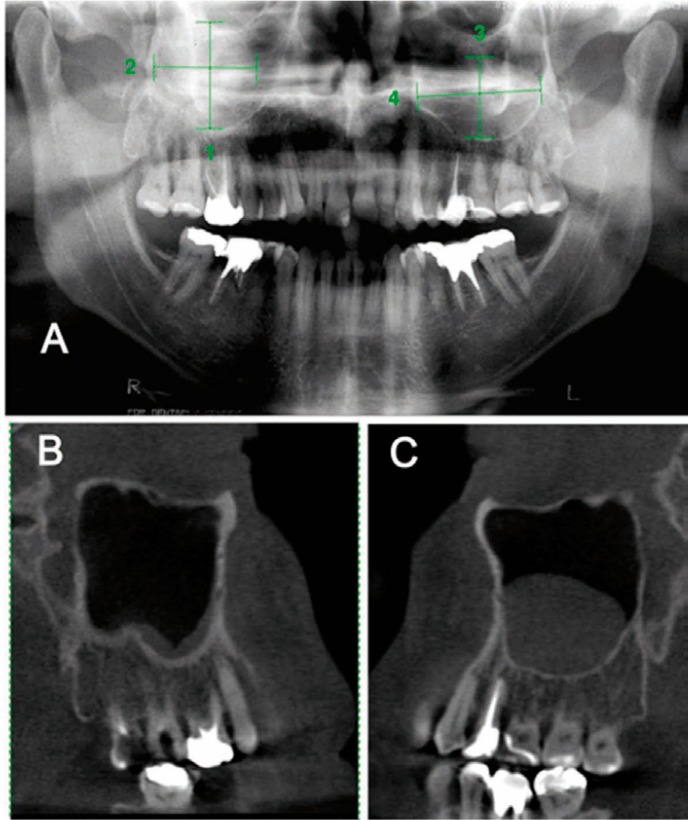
Control panoramic radiograph of bilateral MRCMS A), sagittal CBCT reconstruction of same patient, with no MRCMS in right side B) and MRCMS in left side C).
